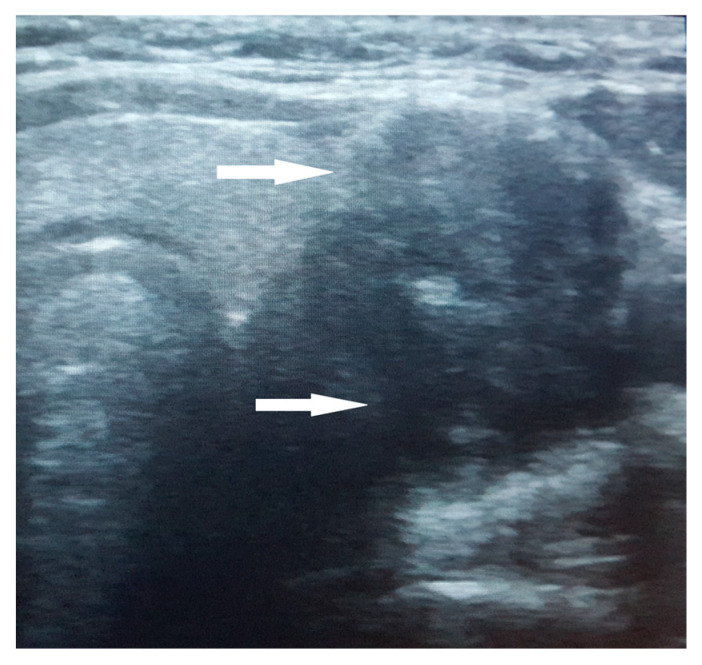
Ultrasound aspect of the large nodule in the left thyroid lobe. The nodule exhibits a non-homogeneous, hypoechogenic structure with poorly defined margins and internal calcifications (arrow).
